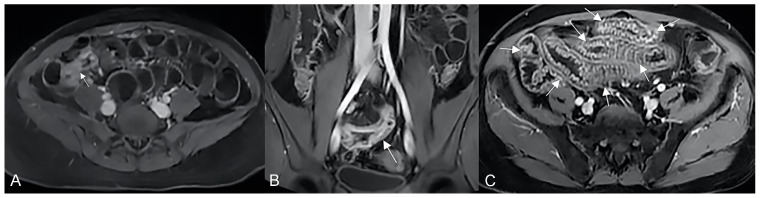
Focal, segmental, or diffuse thickening. (A) Axial T1-weighted after gadolinium injection image shows focal thickening of the distal ileum, shorter than 5 cm in length (arrow), with avid contrast enhancement. (B) Coronal T1-weighted after gadolinium injection image shows segmental thickening of an ileal loop in the pelvis, with 6–40 cm in length (arrow). (C) Axial T1-weighted after gadolinium injection image shows diffuse thickening of a large amount of small bowel loops (>40 cm) (arrows).
